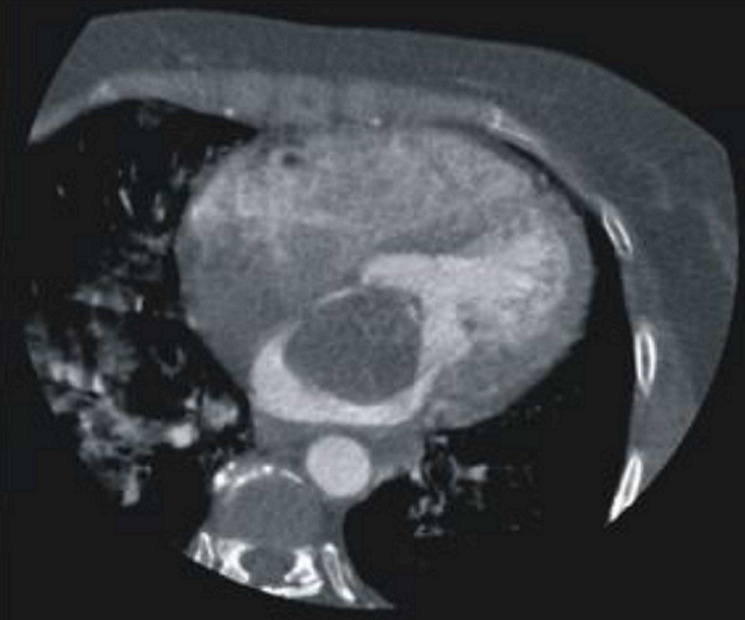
chest computed tomography (CT) scan; a left atrial mass is seen with an attachment to the interatrial septum
